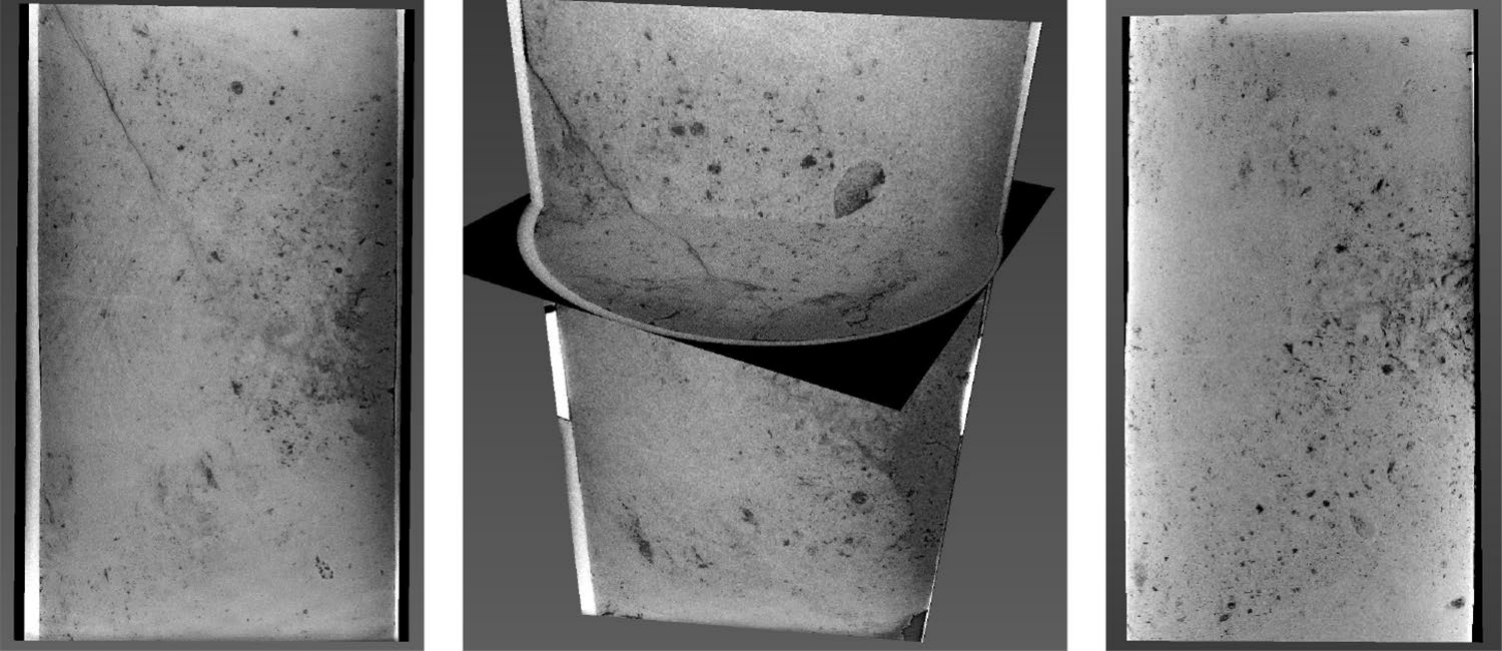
CT scan of C8 post-loading (first two images) and pre-loading (third image). The display is the same as in Fig. 3. The sample size is also the same as that of C23.
